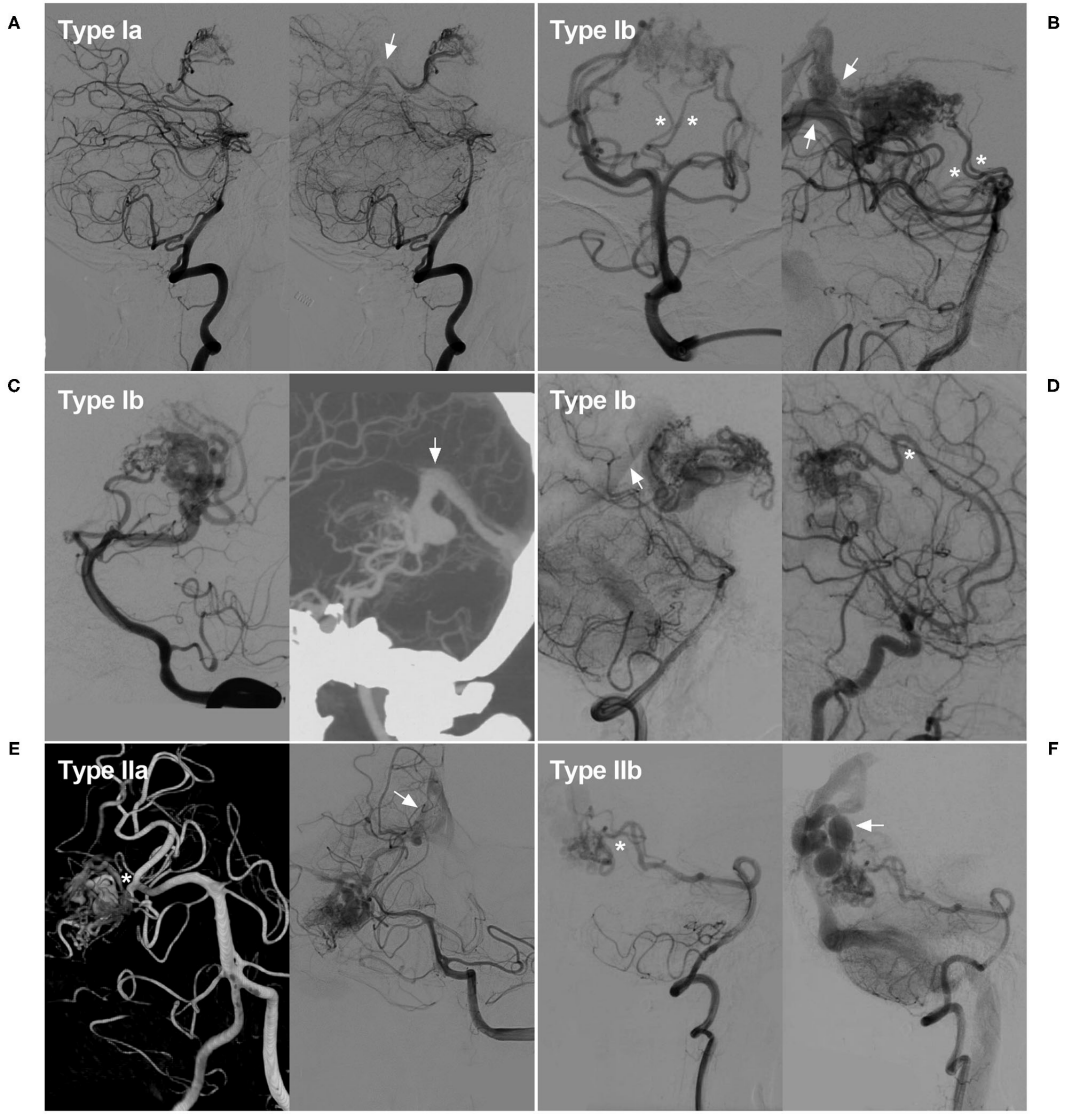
Classification of the PCA-BAVMs. (A) Angiogram of the VA in lateral view shows a Type Ia BAVM supplied by the PchA from the PCA only and drained to the deep venous system (arrow). (B) Angiogram of the VA in anteroposterior (left) and lateral (right) views shows a type Ib BAVM supplied by the PchA (asterisk) and the proximal perforating branches of the PCA (asterisks). Multiple deep venous drainage (arrows) can be observed. (C) Angiogram of the VA in lateral (left) view shows a type Ib BAVM supplied by the PchA and the lateral and medial occipital branches of the PCA. Computed tomography angiography (right) in maximum intensity projection shows the BAVM drains through the deep venous system (arrow). (D) Angiogram of the VA (left) and internal carotid artery (right) in lateral view shows a type Ib BAVM supplied by the PchA and ACA (asterisk). Deep venous drainage (arrow) can be observed. (E) Angiogram of the VA shows a Type IIa BAVM supplied by the temporal branch (asterisk) of the PCA and drained to the deep venous system (arrow). (F) Angiogram of the VA shows a Type IIb BAVM supplied by the medial occipital branch (asterisk) and drained through the cortical veins (arrow). ACA, anterior cerebral artery; BAVM, brain arteriovenous malformation; PCA, posterior cerebral artery; PchA, posterior choroidal artery; VA, vertebral artery.
